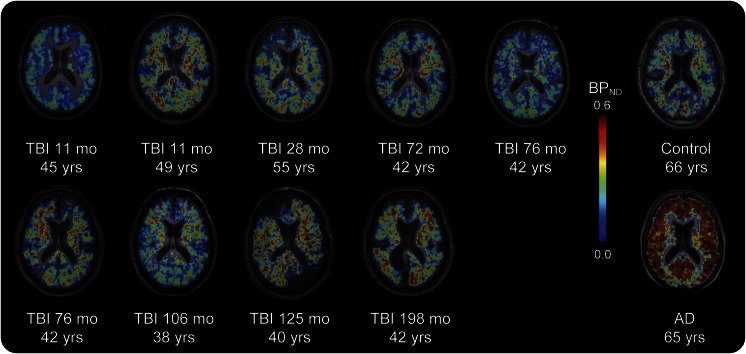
^11^C-PiB binding following TBI Images of axial T1 MRI are superimposed with ^11^C-PiB BP_ND_ maps for all patients with TBI and a representative healthy aged control and a participant with AD. For patients with TBI, the interval in months from the time of TBI to PET scanning and the age in years of each participant at scanning is also shown. AD = Alzheimer disease; BP_ND_ = nondisplaceable binding potential; ^11^C-PiB = ^11^C-Pittsburgh compound B; TBI = traumatic brain injury.
